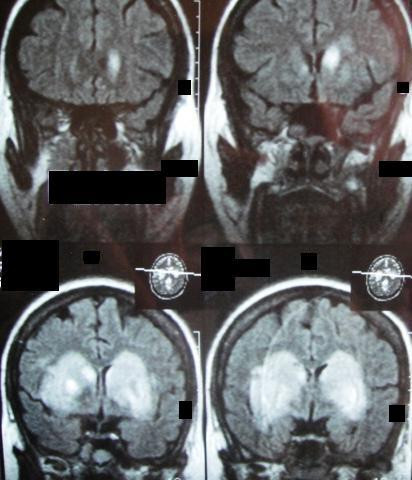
Coronal T2 FLAIR (fluid attenuation inversion recovery) brain MRI film of our patient on the fifth day of hospital stay. Note the bilateral heterogeneous hyper intensities at the right and left corpus striatum. These areas (which were not suppressed on this film) represent ischemic infarction with hemorrhagic transformation.
